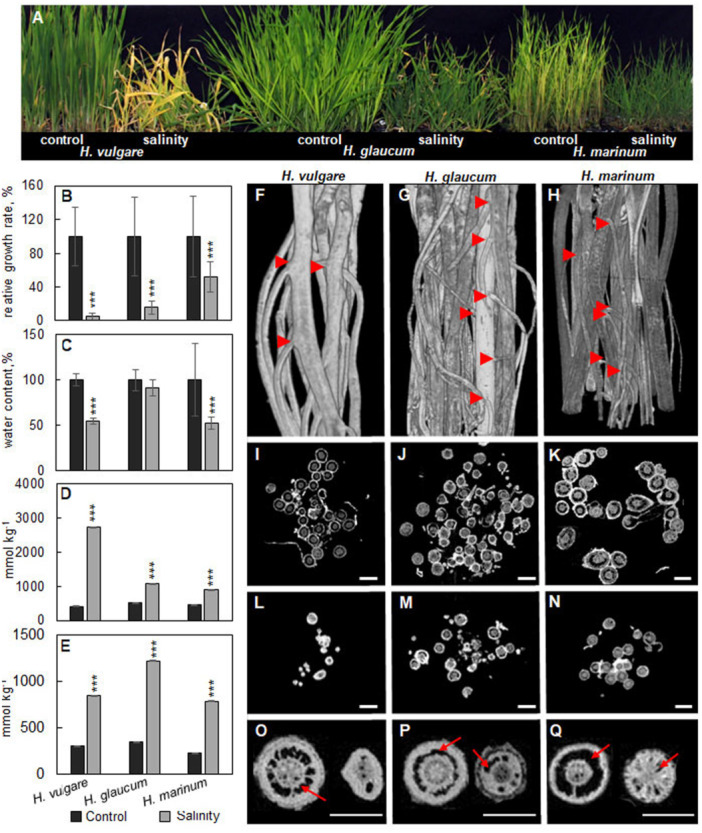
Phenotypic appearance of *Hordeum vulgare*, *H. glaucum,* and *H. marinum* plants grown under control hydroponic and salinity stress conditions. (A) Shoot phenotype; (B) Relative growth rate; (C) Plant water content; (D, E) Osmotic pressure of tissue sap from shoots (D) and roots (E). Data are mean ± SD; *n* = 5, *t* significant (Student's *t*‐test) at: p* < 0.001. (F–Q) Root phenotypes as analysed by Magnetic Resonance Imaging. (F, G) 2D projections of 3D images of salt‐stressed roots of *H. vulgare* (F), *H. glaucum* (G), and *H. marinum* (H). The 3D images are shown in the Supplementary information Movies S1 and S2. Red triangles point to root branches. (I–N) Virtual cross sections of control (I–K) and salt‐stressed roots (L–N) of *H. vulgare* (I, L), *H. glaucum* (J, M), and *H. marinum* (K, N). (O–Q) Virtual cross sections of individual control (left) and salt‐stressed roots (right) of *H. vulgare* (O), *H. glaucum* (P), and *H. marinum* (Q). Bars, 1 mm in (I–N), 500 µm in (O–Q).
